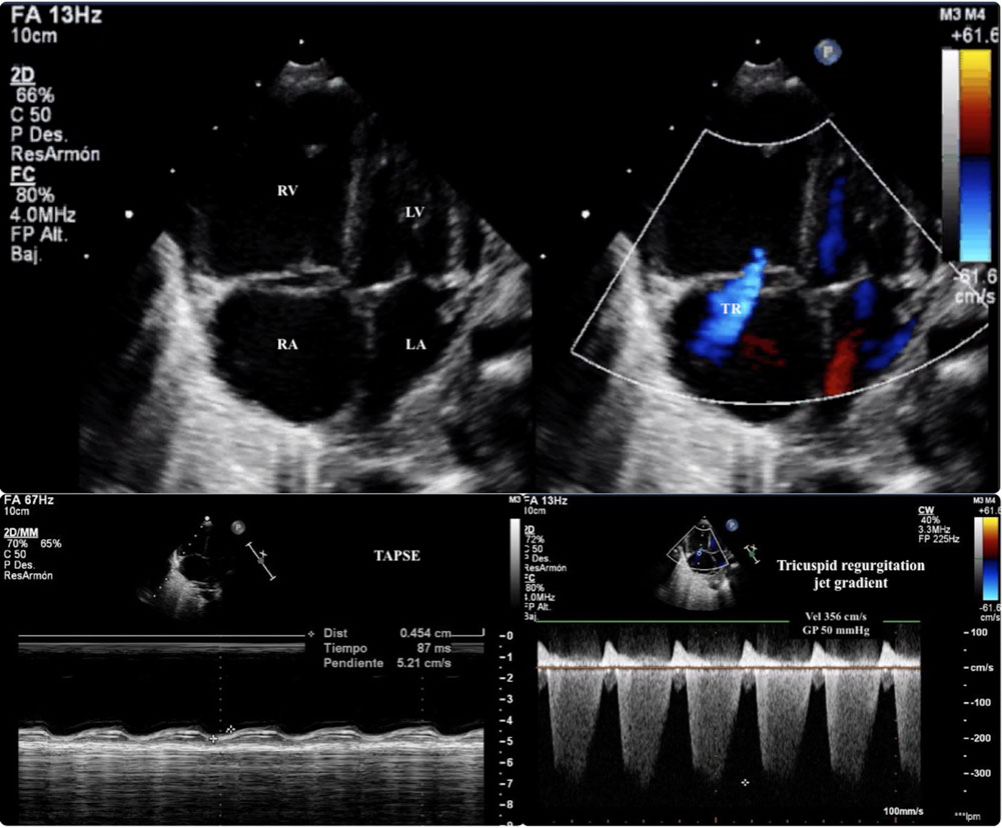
Echocardiography: The upper panel is an apical 4-chambers view that shows a dilated RV with severe tricuspid regurgitation and interauricular septum domed to the LA. The estimated systolic pulmonary arterial pressure that was 70 mmHg (50 mmHg of the TRJG + 20 mmHg of estimated RA pressure; the bottom right-sided panel), respect a systolic arterial pressure of 60 mmHg at the moment of the echocardiography. All the above constitute echocardiographic signs of supra-systemic PH). The bottom leftsided panel is a M-Mode in an apical 4-chambers view that shows a severely depressed RV systolic function (TAPSE 4.5 mm). RV (right ventricle); LV (left ventricle); RA (right atrium); LA (left atrium); TR (tricuspid regurgitation jet); TRJG (tricuspid regurgitation jet gradient); TAPSE (Tricuspid annular plane systolic excursion).
